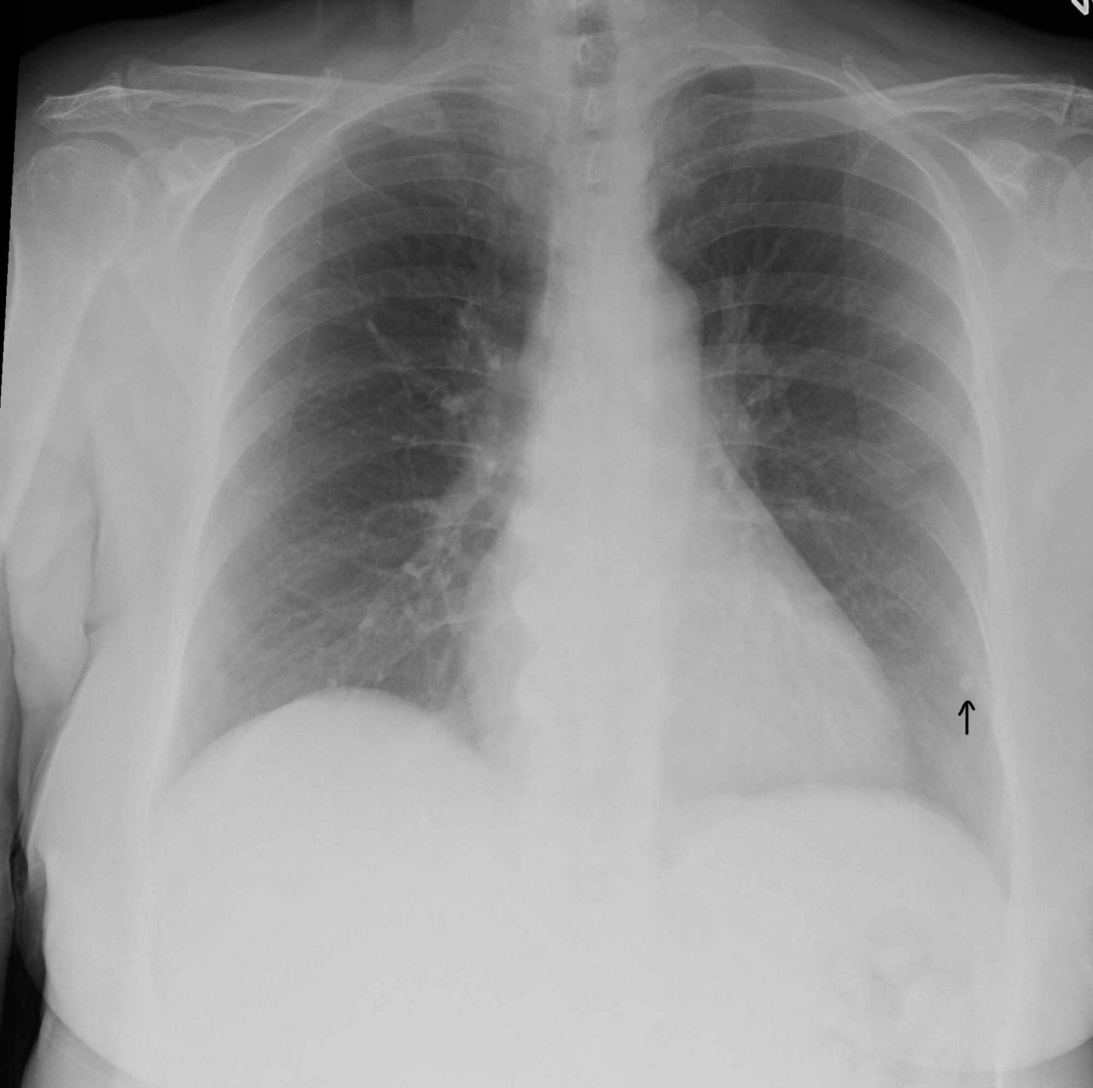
Chest radiograph demonstrating stable calcified granuloma (arrow)
